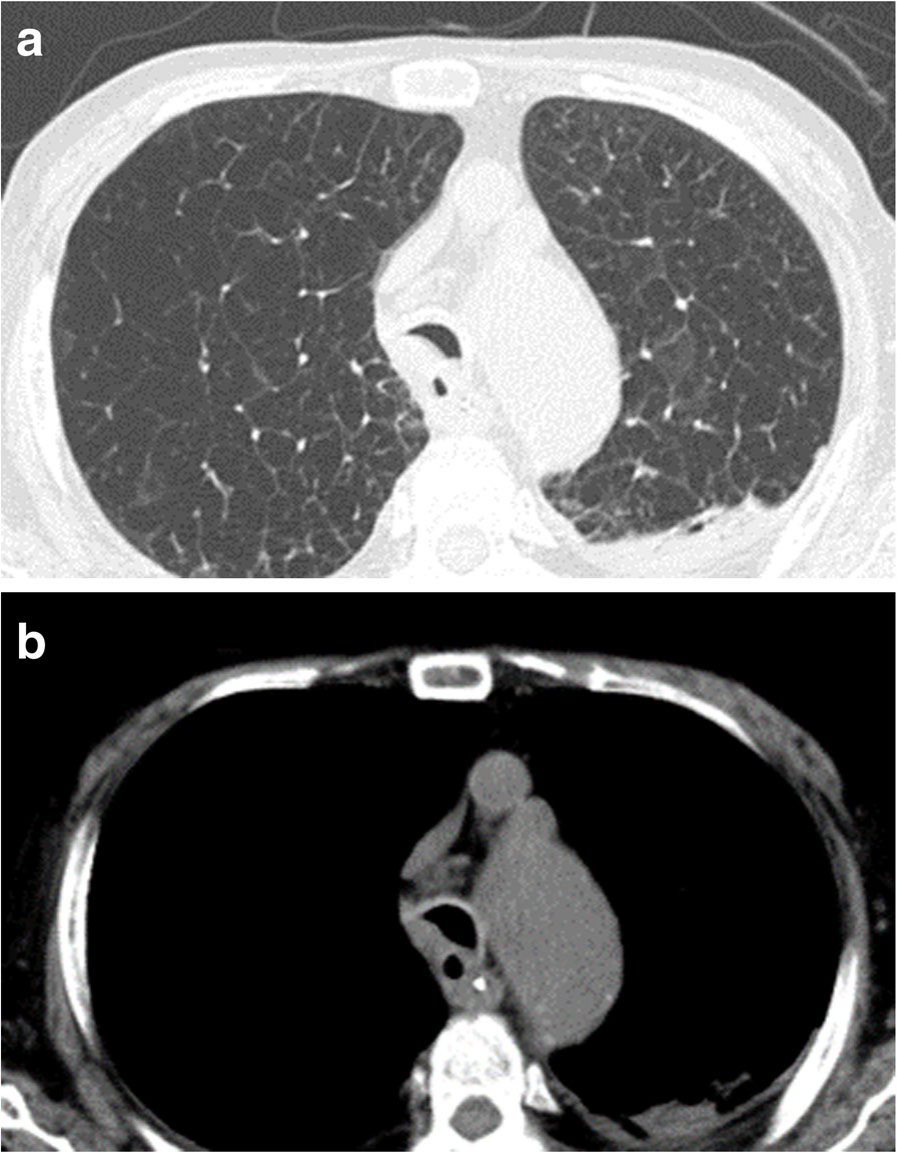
Postoperative chest computed tomography (CT). a Expansion of the stenotic area on chest CT at 6 months postoperatively. b Scars covering the dorsal side of the membranous portion of the bronchial tube at 6 months postoperatively
